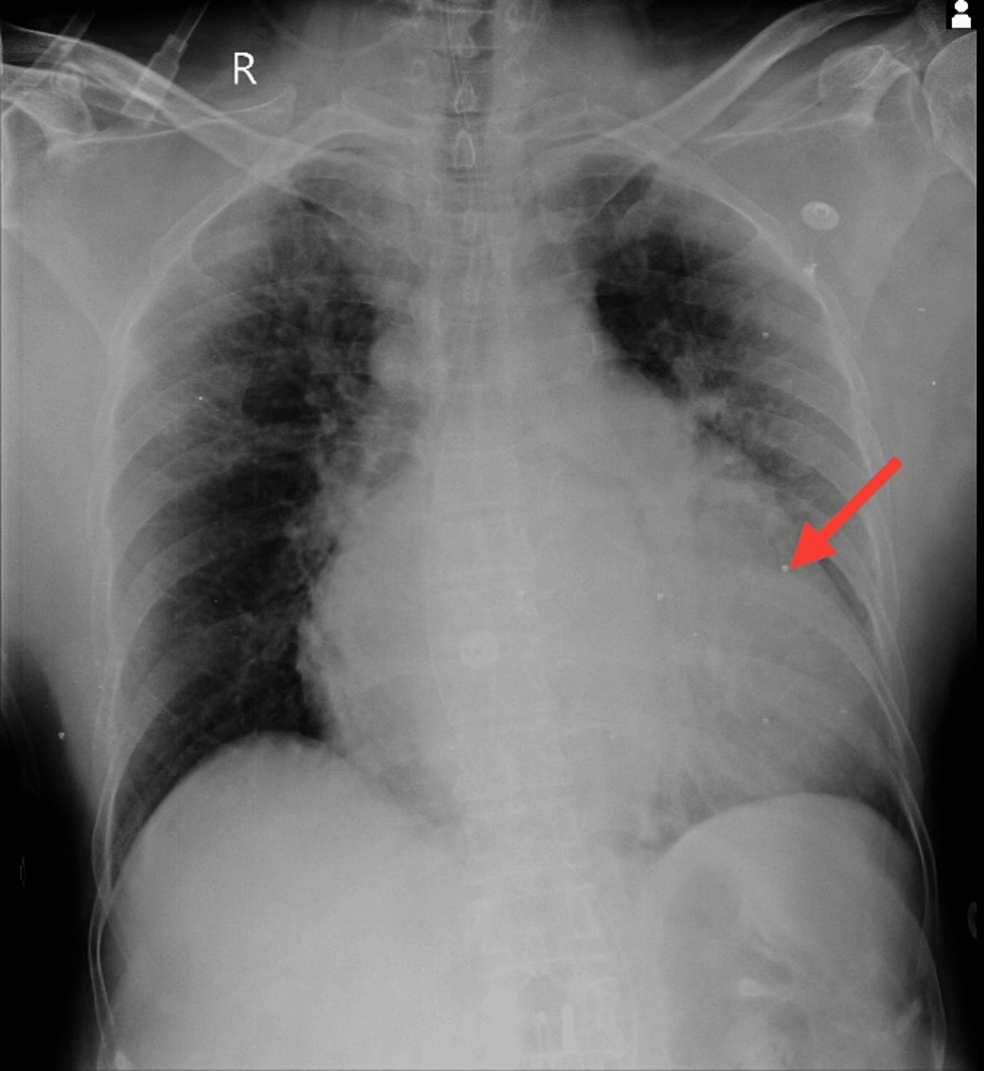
Chest X-ray eliciting cardiomegaly The red arrow shows cardiomegaly.
